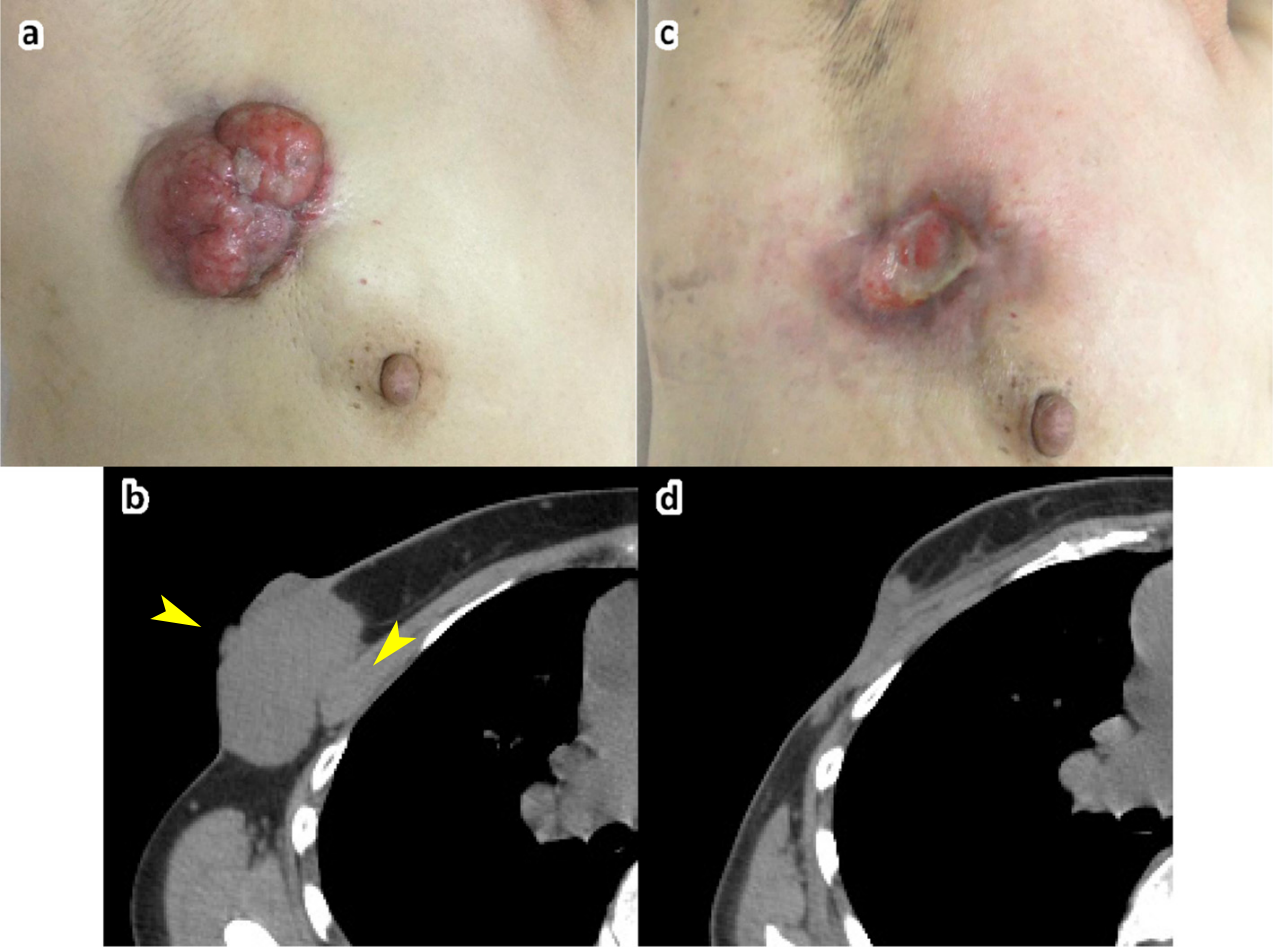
Case 2.a: Macroscopic image of the right breast at the initial visit. b: Computed tomography (CT) findings at the initial visit. CT revealed a mass with infiltration of the pectoralis major muscle (arrowheads). c: Macroscopic image of the right breast before surgical treatment. d: CT findings before surgical treatment.
